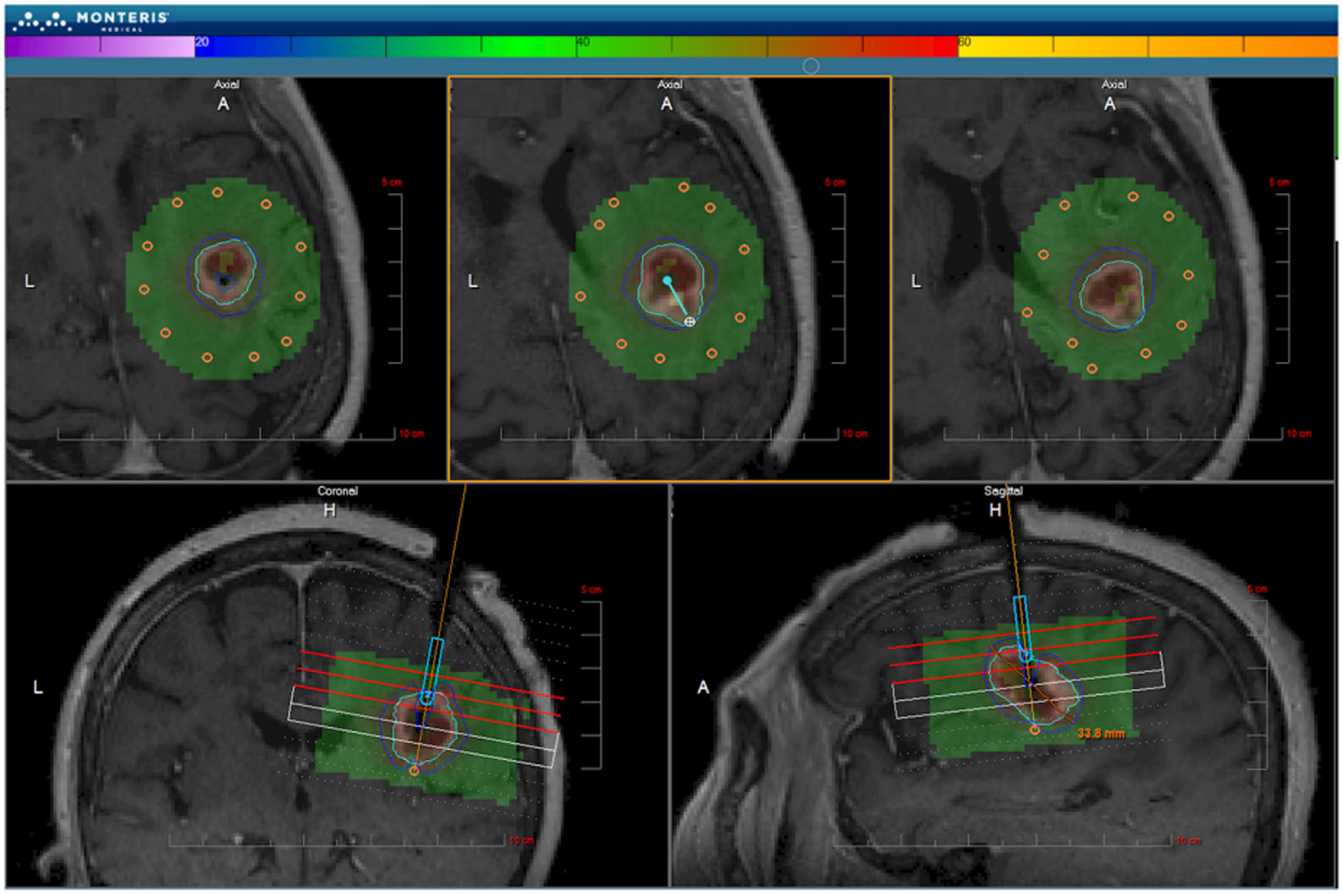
NeuroBlate software. The M*Vision software allows the target volume to be contoured slice-by-slice, thermal dose threshold lines are calculated, and the laser probe trajectory can be planned. After planning, the software executes the treatment allowing the user to monitor real-time thermal imaging. Laser adjustments can be made during treatment. Once the ablation is complete, MR images are obtained and compared with thermal dose thresholds. ©Monteris Medical. Used by permission.
